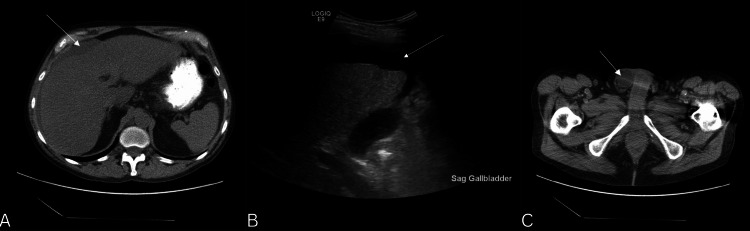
Preoperative axial CT scan (A and C) and preoperative sagittal right upper quadrant ultrasound (B) of a 62-year-old male, with ascites from alcoholic cirrhosis. White arrows demonstrate ascites (A and B) and a right inguinal hernia (C).
